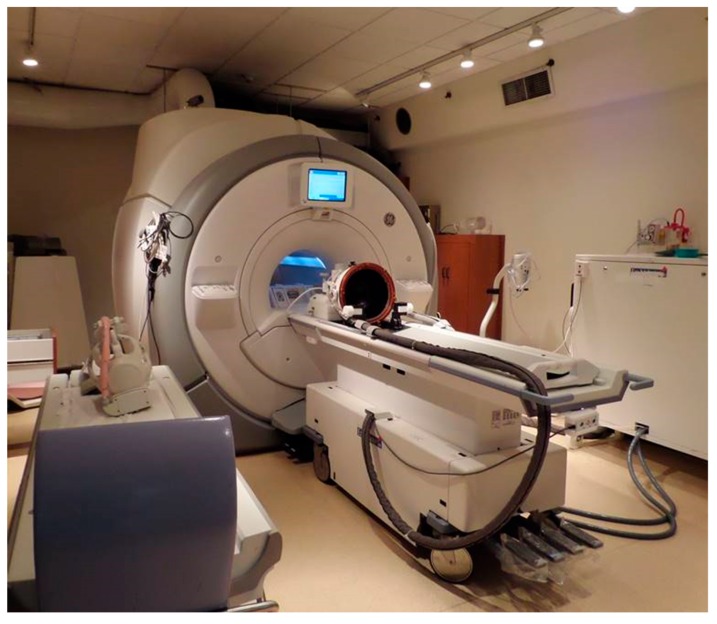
An ExAblate Neuro (InSightec, Haifa, Israel) MRgFUS transducer helmet on an MRI table (located at Sunnybrook hospital, Toronto, Canada).
